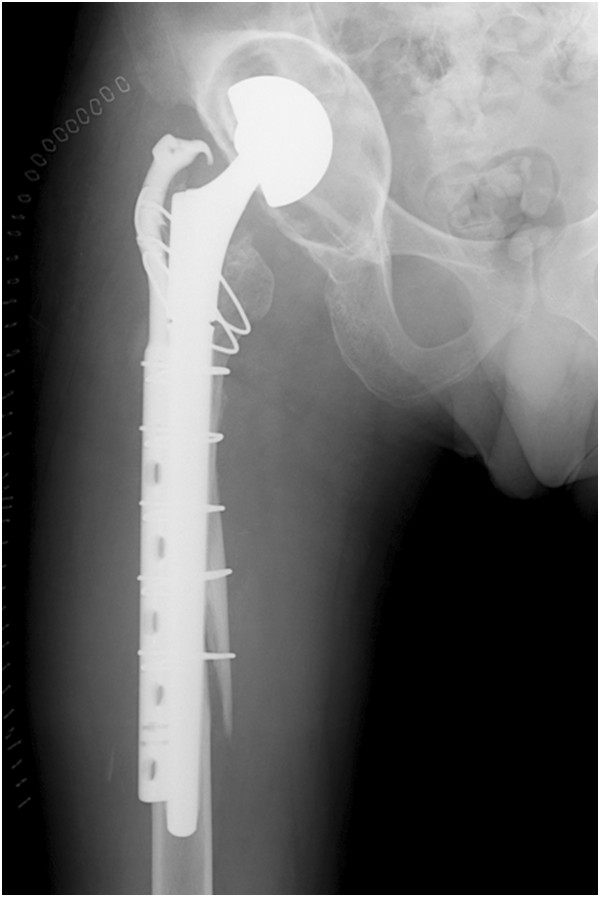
X-ray showing new hemiarthroplasty of the hip joint with a modular neck and long stem.
